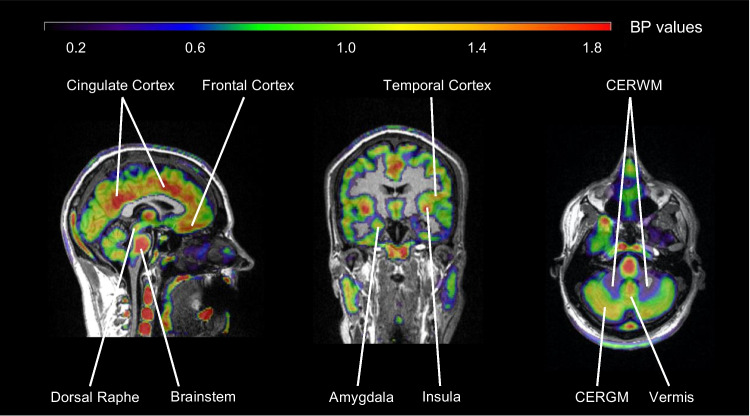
Parametric image of BP values estimated with LREF_CERWM_. BP image is overlaid on the T1 MRI of the participant (CERWM = cerebellum white matter; CERGM = cerebellum grey matter)
